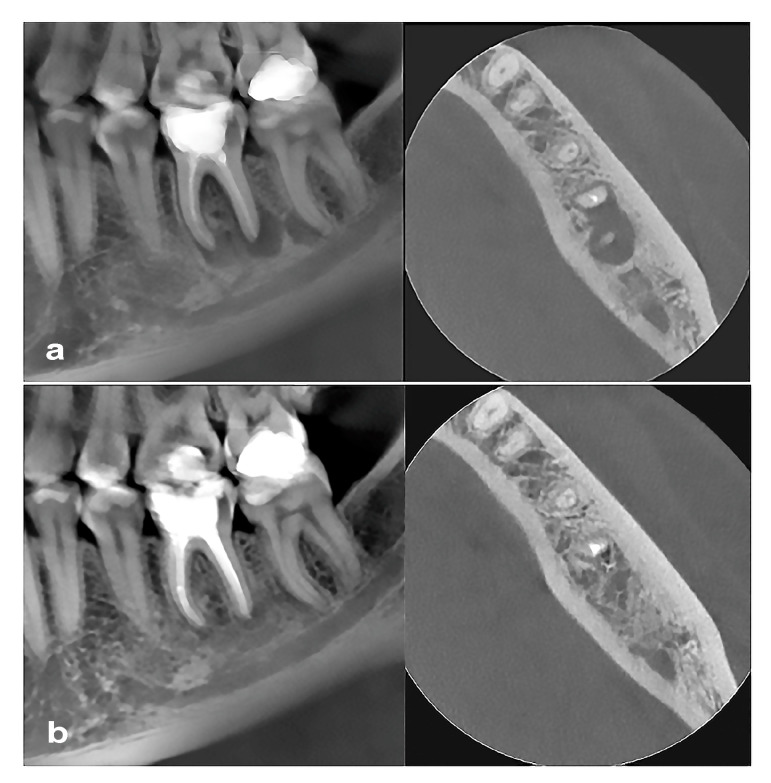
Representative CBCT of a lower third molar with inadequate root canal filling and large periapical lesion (a), and at 1-year follow-up (b) showing significant healing of the lesion after root canal filling with BioRoot RCS.
